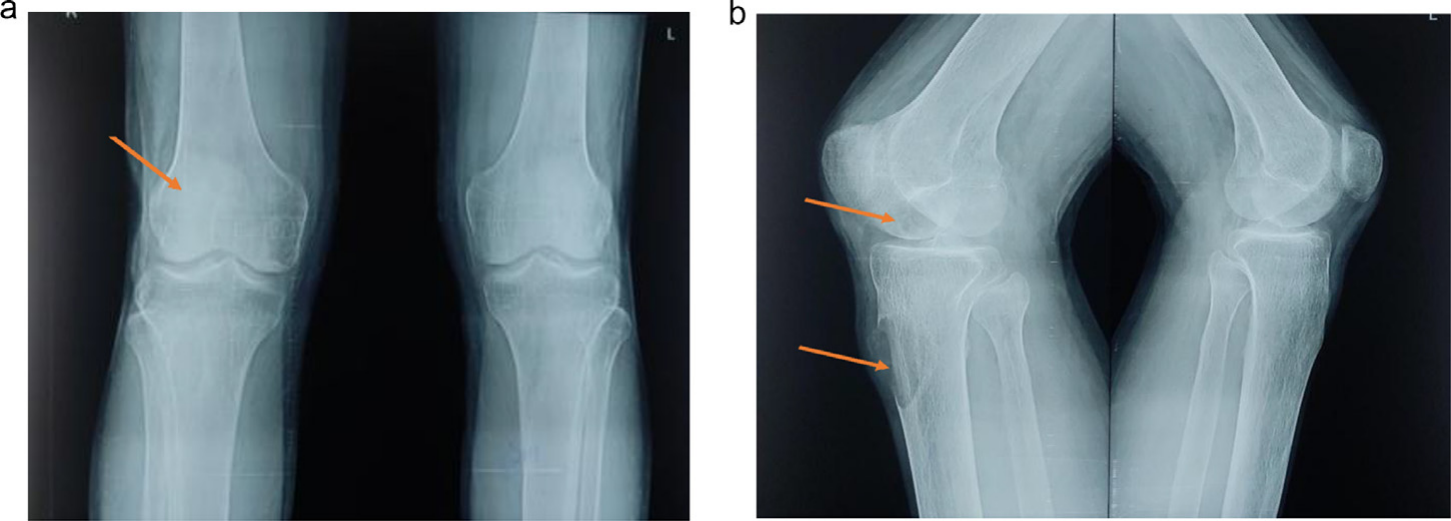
Simple radiograph of bilateral knee joints presenting a lesion in the right knee on Anteroposterior view while the left knee is normal. (B) A well-defined osteolytic geographic lesion at the epiphysis of the medial femoral condyle extending up to the subarticular region and an eccentric lytic lesion at the proximal end of the tibia showing cystic changes with decreased bone density.
